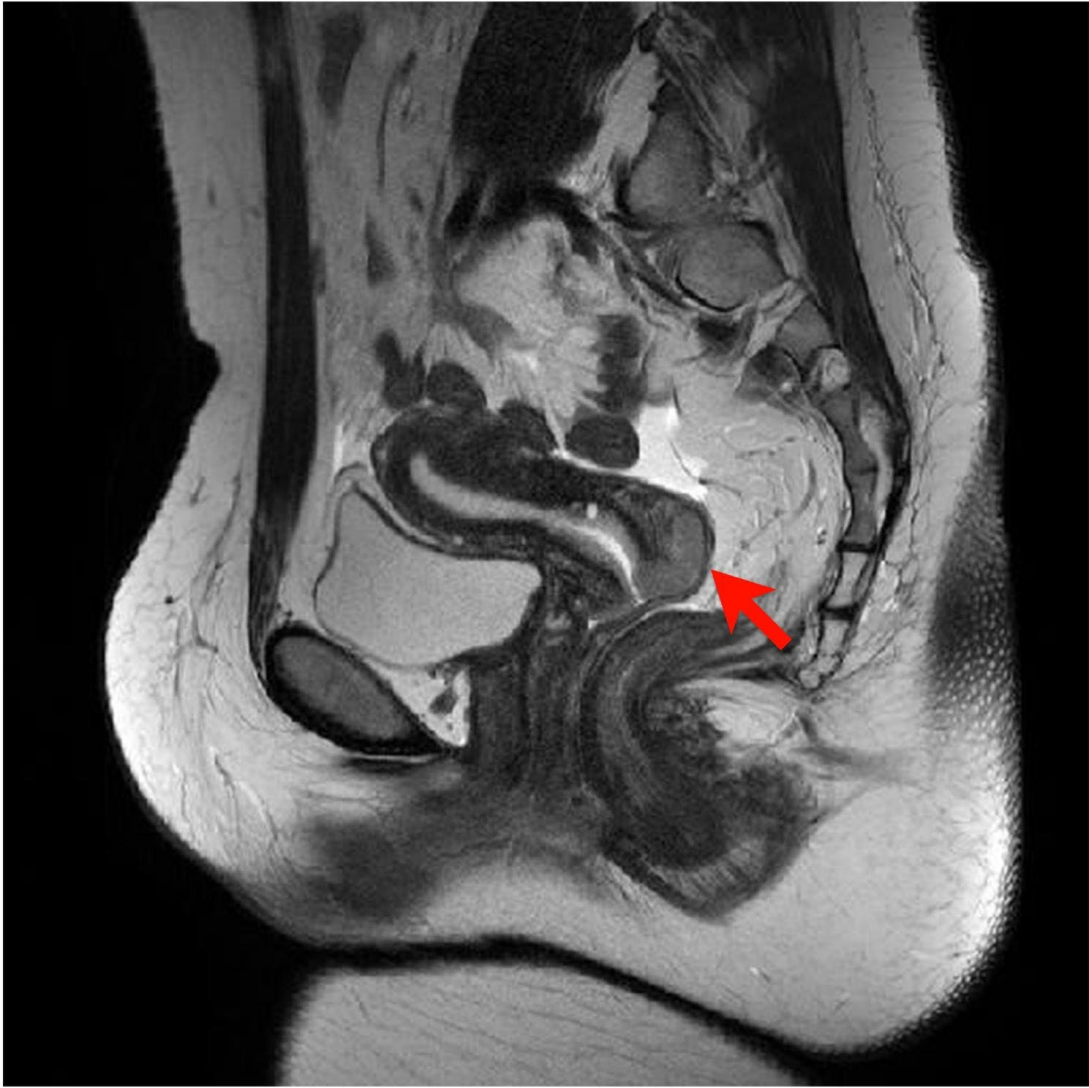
Magnetic resonance imaging. An exophytic mass is more prominent in the T2-weighted image in the posterior uterine cervix (arrow)
